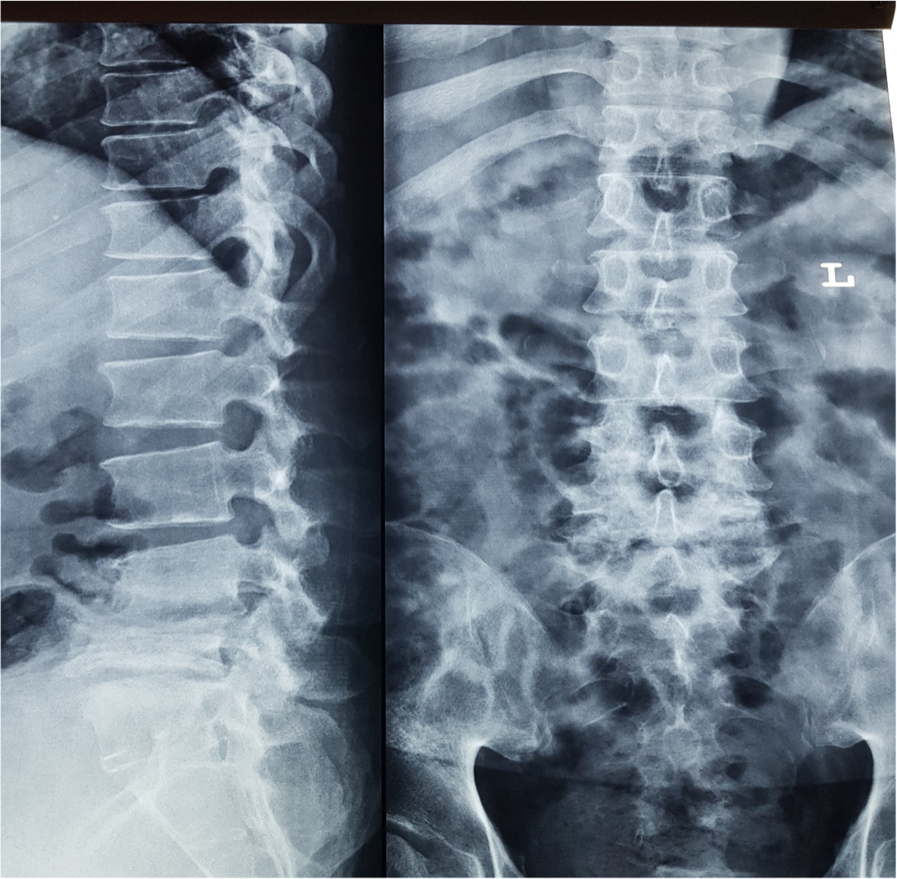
Lumbosacral radiograph showing irregularities of the L4, L5, and S1 endplate with reduction in L4/L5 and L5/S1 intervertebral discs space and L5 vertebral body
